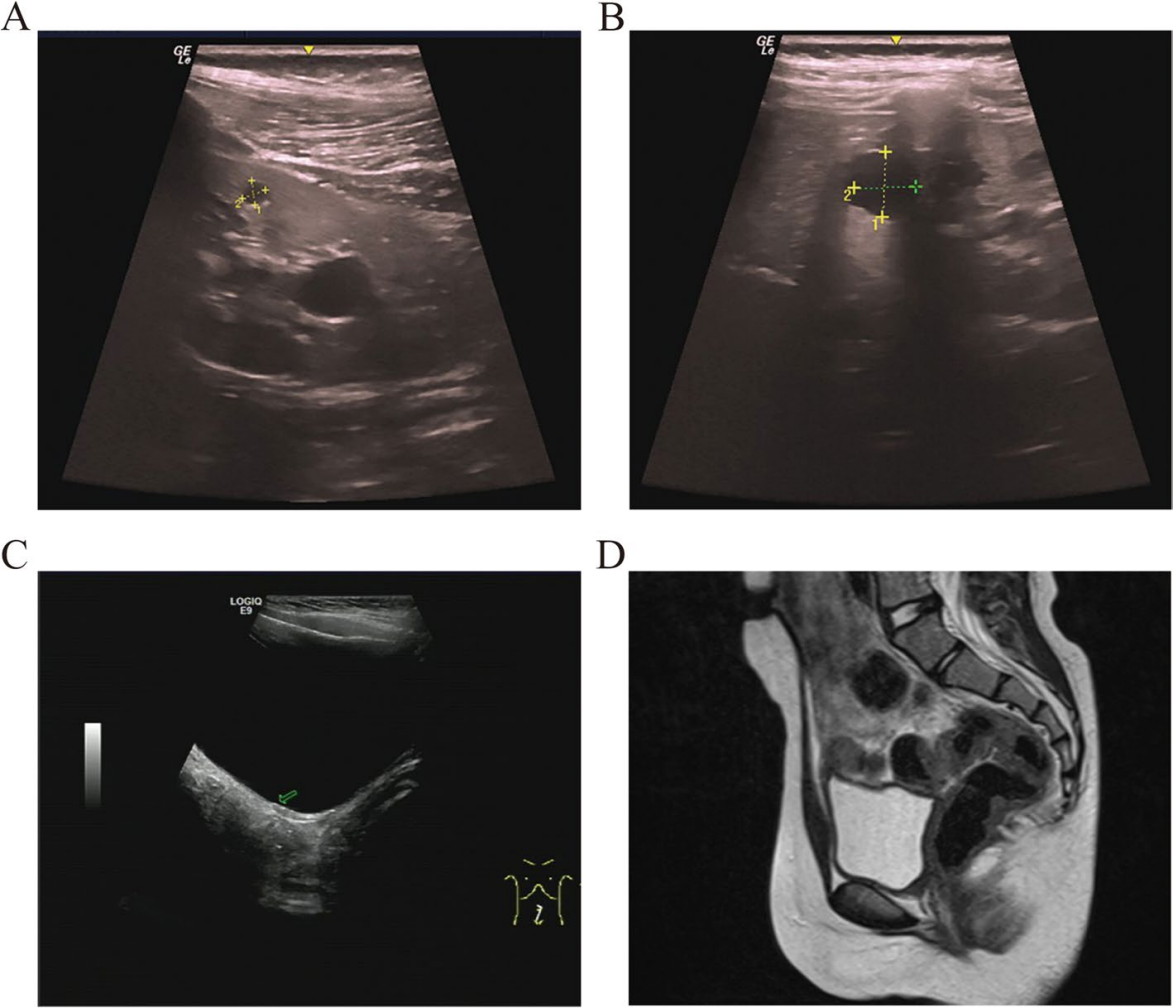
The pelvic ultrasound and MRI of case 5. A, B Ultrasonography of the kidneys. Numerous cysts are scattered in the kidney. C The pelvic ultrasound of case 5. D The pelvic MRI of case 5
